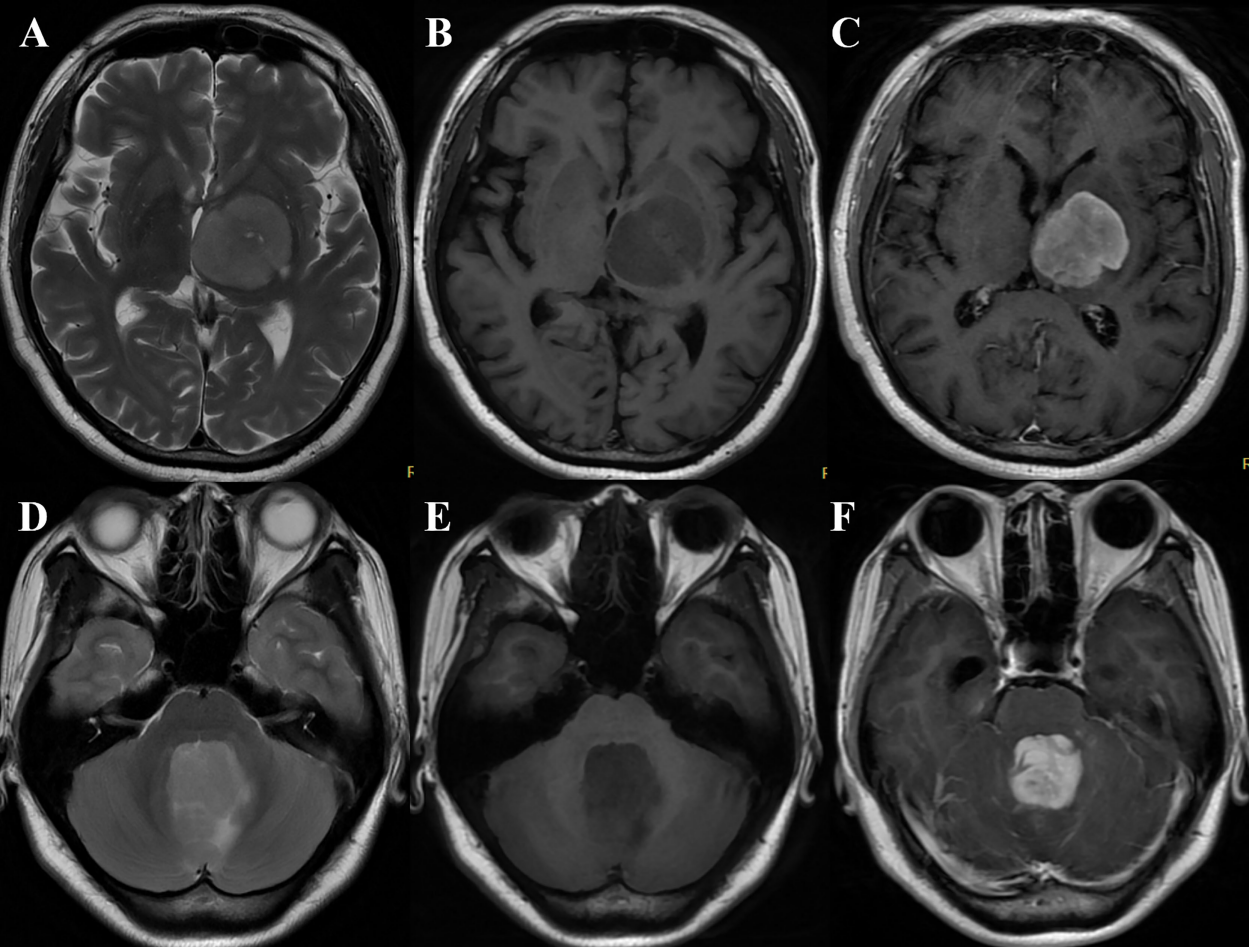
(A–C) A 60-year-old woman with primary central nervous system lymphoma (PCNSL) presented with right hemiparesis for 3 months. MRI demonstrated the lesion was located in the left basal ganglia and thalamus with slight hyperintensity on T_2_WI (A), hypointensity on T_1_WI (B), and marked heterogeneous enhancement on T_1_CE imaging (C) (take gray matter for reference). The quantitative parameters showed that rT_1_, rT_2_, T_2_/T_1_, and rT_1_CE were 0.83, 1.34, 1.62, and 1.23, respectively. The case was correctly diagnosed as PCNSL by models 1, 2, 4, 5, and 6 and radiologist B while wrongly classified as glioblastoma (GBM) by radiologist (A). (D–F) A 23-year-old woman with GBM presented with nausea and vomiting for 2 months. MRI showed a vermis lesion with slight hyperintensity on T2WI (D), hypointensity on T1WI (E), and obvious homogeneous enhancement on T1CE imaging (F) (take gray matter for reference). The quantitative parameters showed that rT_1_, rT_2_, T_2_/T_1_, and rT_1_CE were 0.57, 1.91, 3.35, and 2.73, respectively. The case was correctly diagnosed as GBM by models 1, 2, 4, and 5 while wrongly classified as PCNSL by the two radiologists.
